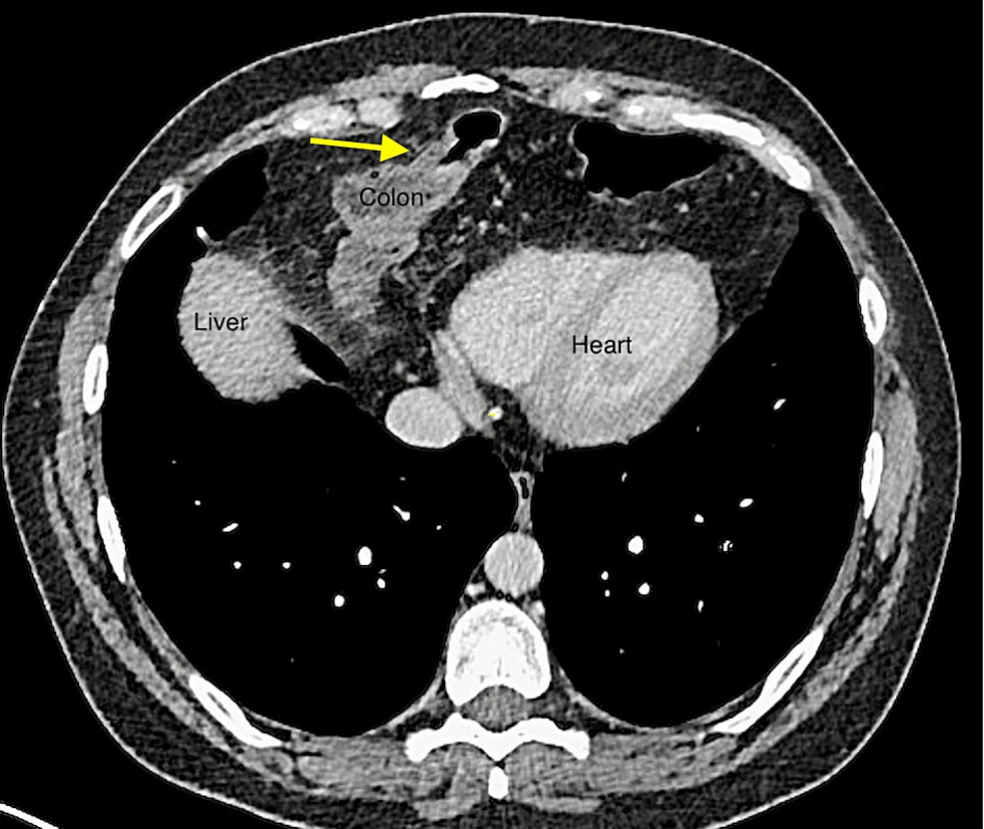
Axial view of abdominal and thoracic CT scan displaying transverse colon and greater omentum in the anterior mediastinum (yellow arrow)
